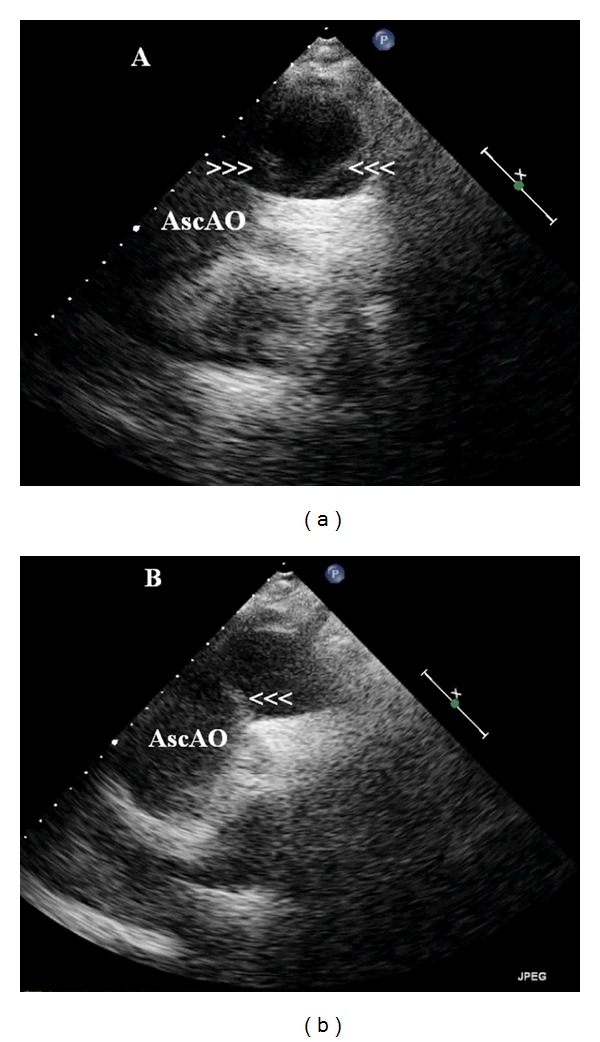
Transthoracic echocardiogram in suprasternal view showing dissection flap in ascending aorta extending up to arch dividing the aorta into a large false lumen and a small true lumen (arrowheads) in a patient with ascending aortic dissection. AscAo: ascending aorta.
